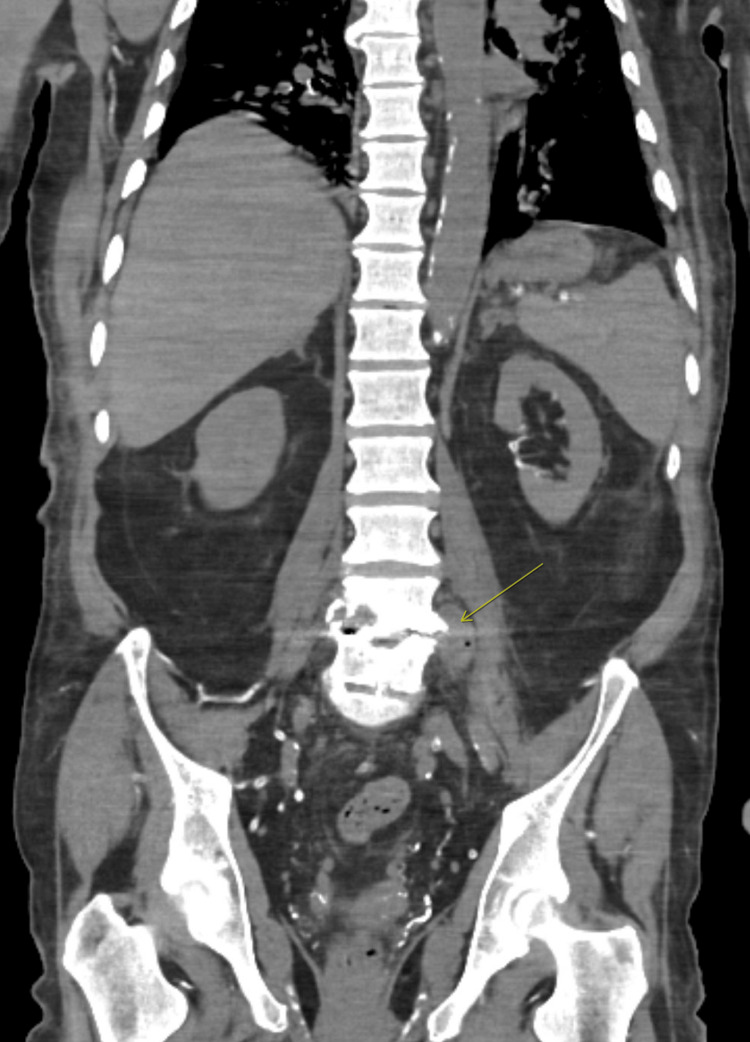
Coronal CT of the abdomen and pelvis without IV contrast demonstrating a left paraspinal abscess (yellow arrow)
